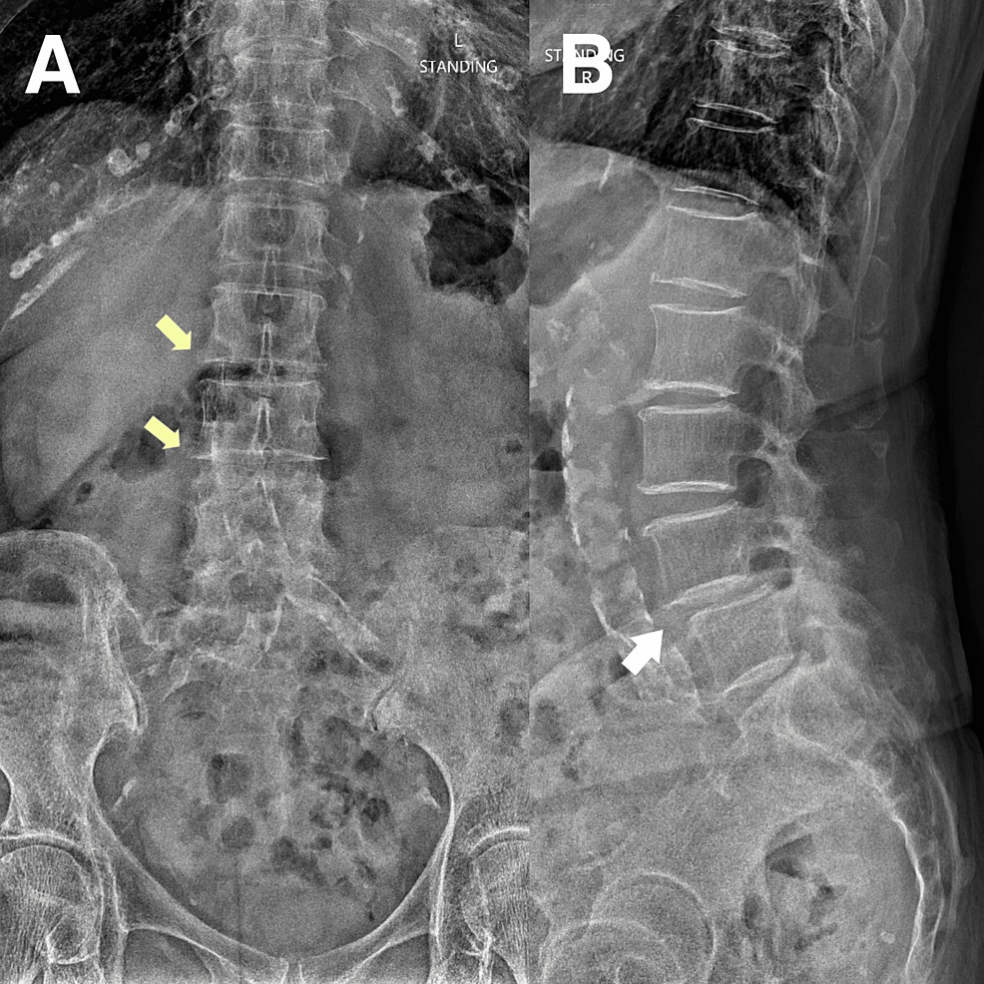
Lumbar radiography A) Anteroposterior view: Marginal osteophytes noted along the lumbar spine suggestive of spondylosis. B) Lateral view: Grade I spondylolisthesis noted at L4/5 and L5/S1. Decreased disc spaces noted from L4-S1 levels suggestive of disc degeneration.
